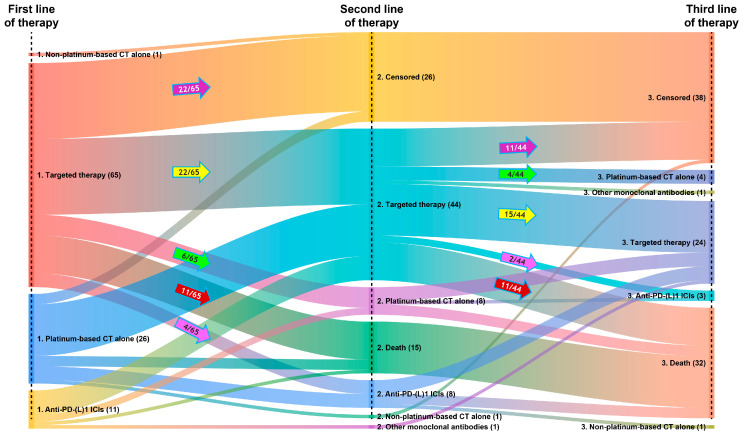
Treatment sequences (first to third line of therapy) for all patients receiving at least one line of SACT (*N* = 103). The visual thickness of the horizontal lines reflects the number of patients following a specific treatment sequence across the first, second, and third line of therapy. In addition, the colored arrows provide specific data on treatment sequences for patients receiving targeted therapy at first or second line of therapy, as follows: purple arrows, targeted therapy followed by censorship; yellow arrows, targeted therapy followed by targeted therapy; green arrows, targeted therapy followed by platinum-based CT alone; red arrows, targeted therapy followed by death; and pink arrows, targeted therapy followed by anti-PD-(L)1 ICIs. Abbreviations: CT, chemotherapy; ICI, immune checkpoint inhibitor; PD-(L)1, programmed death-(ligand) 1.
